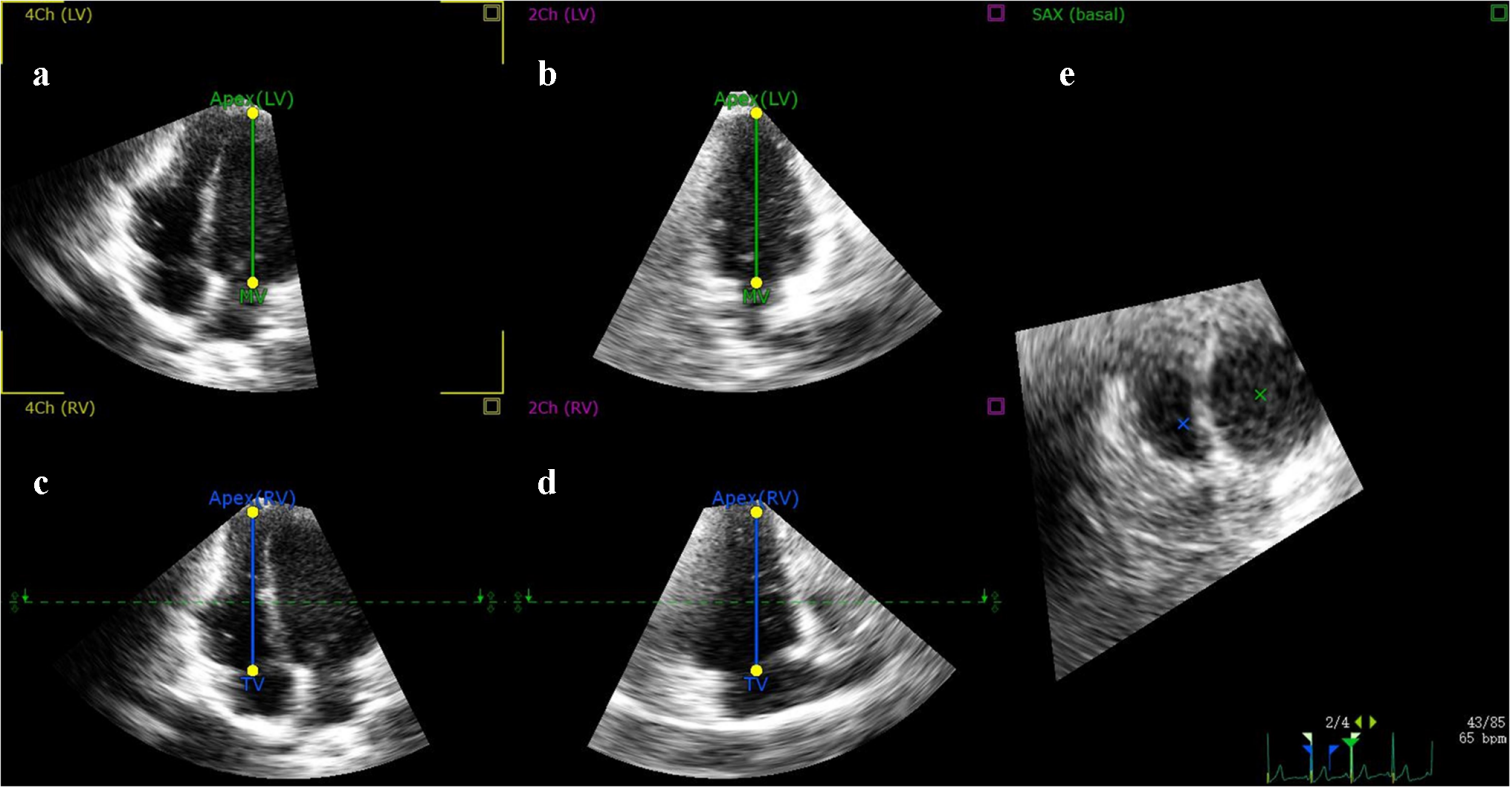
The software enables automatic generation of multiplanar reformations from three-dimensional echocardiography (3DE), depicting the right ventricle of a 6-year-old boy diagnosed with noncompaction cardiomyopathy. When necessary, the operator edited the initial landmarks—including the left ventricular and right ventricular (RV) apex locations, along with the mitral and tricuspid annulus hinge points—using the following standard views: a apical four-chamber; b left ventricular two-chamber; c RV-focused apical four-chamber; d RV inflow-outflow; and e RV short-axis
